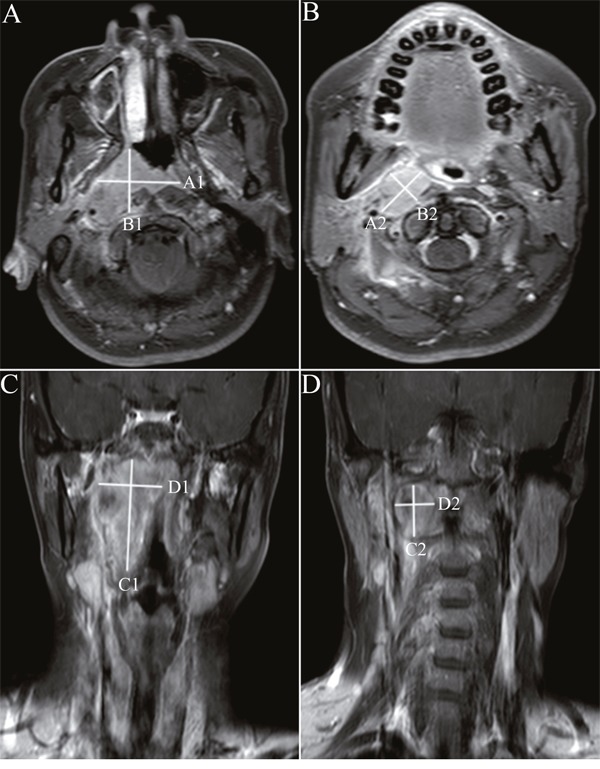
Unidimensional and bidimensional measurements of nasopharyngeal carcinoma in axial and coronal T1-weighted postcontrast MR images Notes: Ax-UDM was obtained by summation of maximum diameter of the primary tumor A. and retropharyngeal nodes B. in the largest axial slice. Ax-BDM was obtained by summation of the products of the Ax-UDM and the greatest measurement perpendicular to it (A) and retropharyngeal nodes (B). Cor-UDM and Cor-BDM of primary tumor C. and retropharyngeal nodes D. were obtained by the same measurements in the largest coronal slice. Ax-UDM (cm) =A1+A2; Ax-BDM (cm^2^) =A1×B1+A2×B2; Cor-UDM (cm) =C1+C2; Cor-BDM (cm^2^) =C1×D1+C2×D2 Abbreviations: Ax-UDM: unidimensional measurements in axial planes; Ax-BDM: bidimensional measurements in axial planes; Cor- UDM: unidimensional measurements in coronal planes; Cor-BDM: bidimensional measurements in coronal planes.
